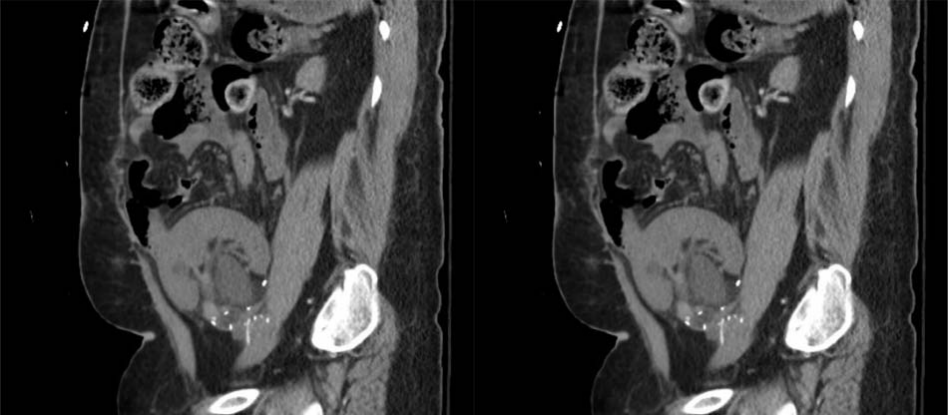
Coronal view of the CT abdomen pelvis without contrast showing hydronephrosis of the donor’s kidney.
